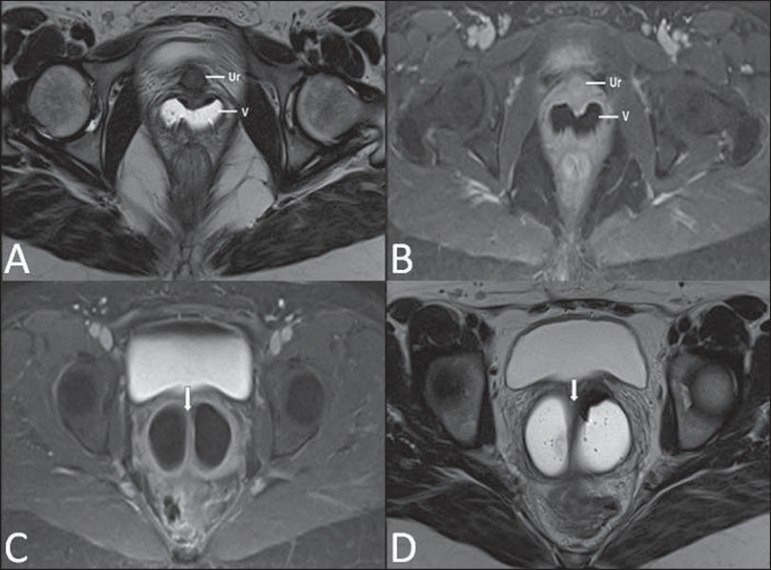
Longitudinal vaginal septum. Contrast-enhanced MRI T2- weighted
